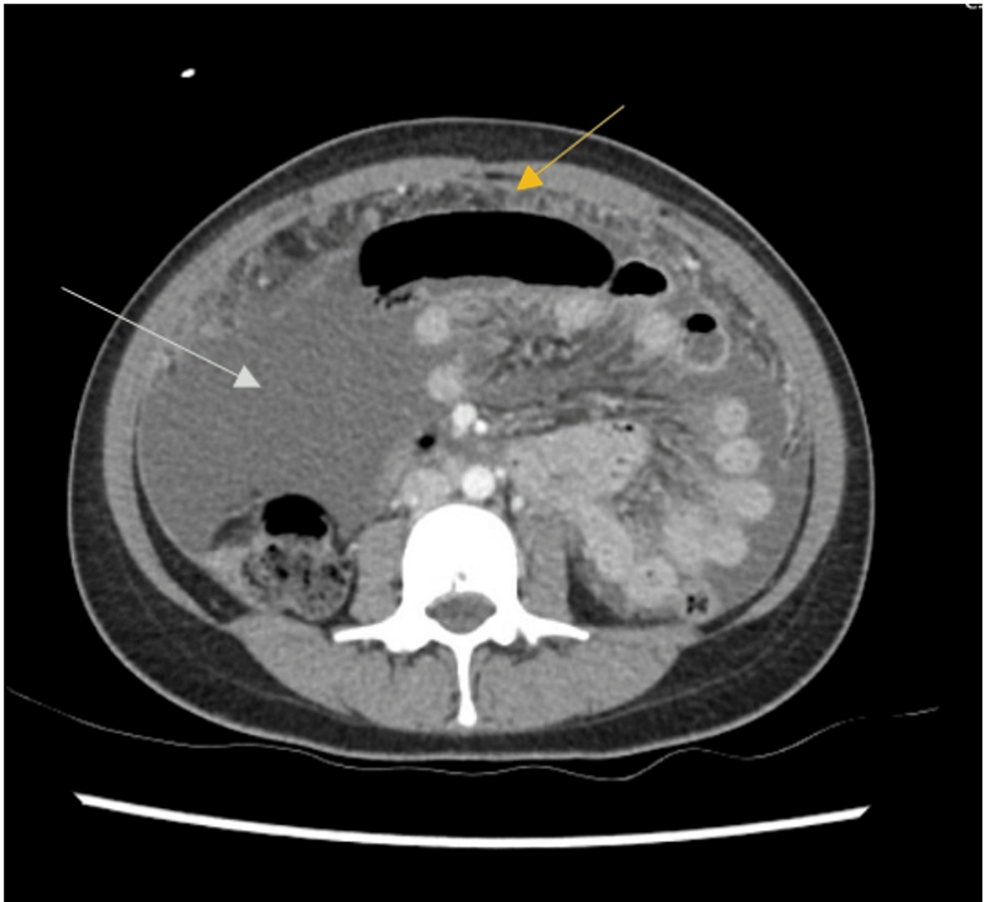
Axial computed tomography scan demonstrating large volume intra-abdominal free fluid (white arrow) with diffuse peritoneal and omental thickening and enhancement (yellow arrow)
